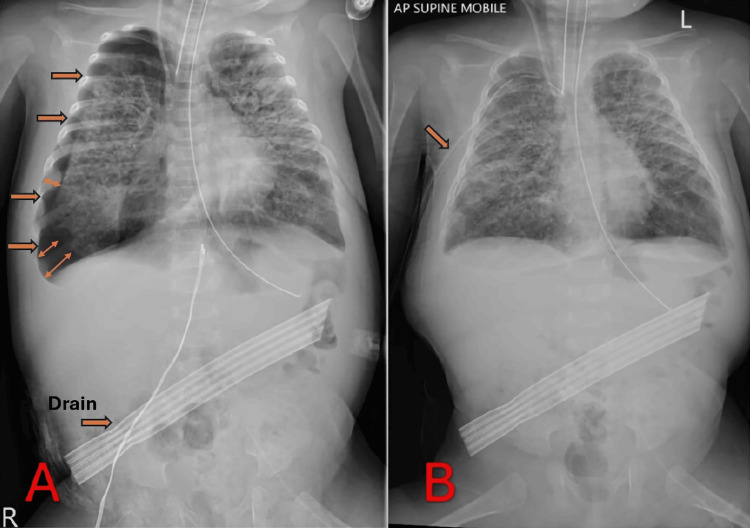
“Intervention” Radiographs Following Drain Placement and Pneumothorax Management A: Supine AP chest and abdominal X-ray after drain insertion, revealing a new right-sided pneumothorax (“the space with air”); B: Follow-up radiograph after chest tube placement, demonstrating re-expansion of the right lung with no obvious residual intraperitoneal air.
